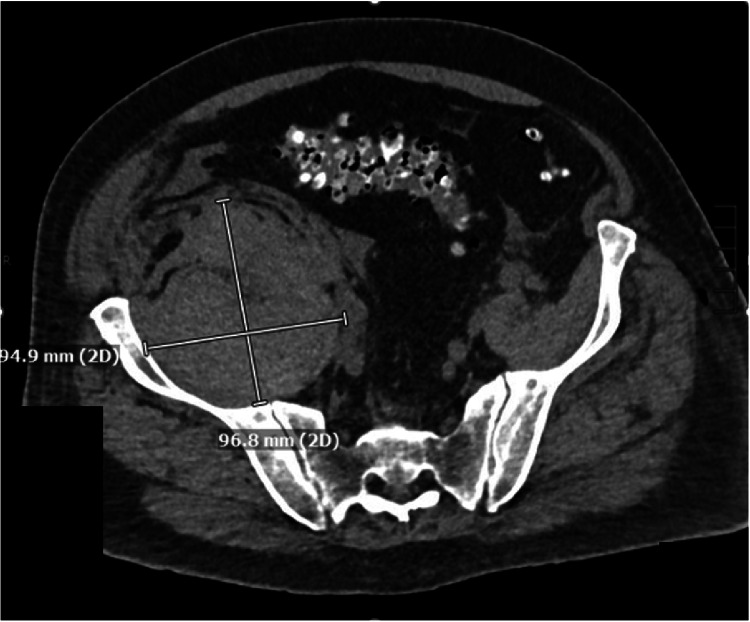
Computed tomography (CT) scan. A right-sided 96.8 mm by 94.9 mm spontaneous retroperitoneal hematoma is visible.
